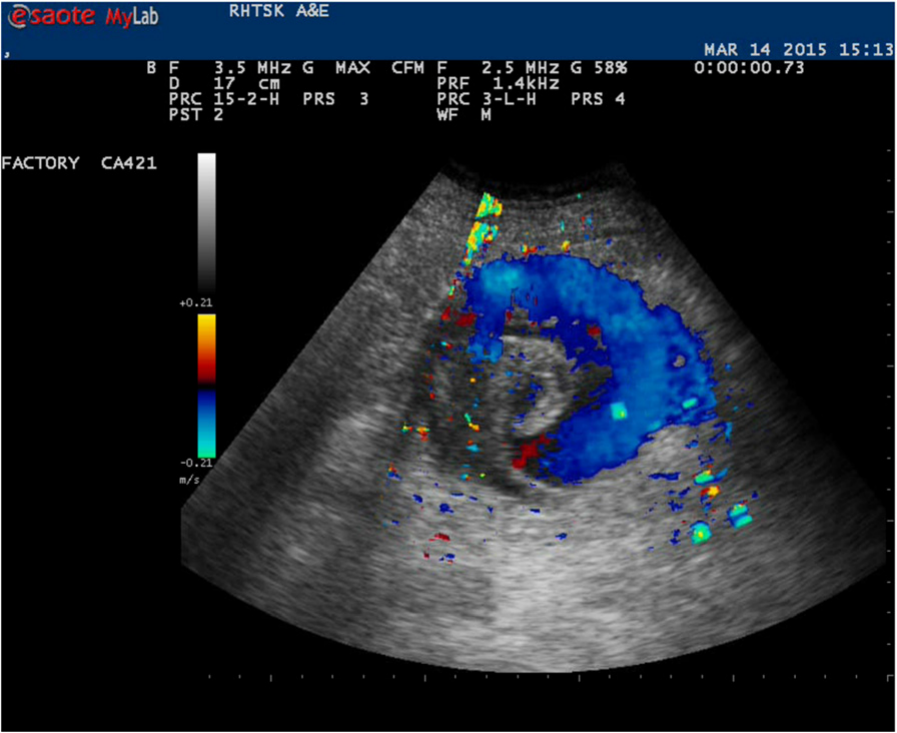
Strong Doppler signal was detected inside the lesion, signifying strong blood flow inside
